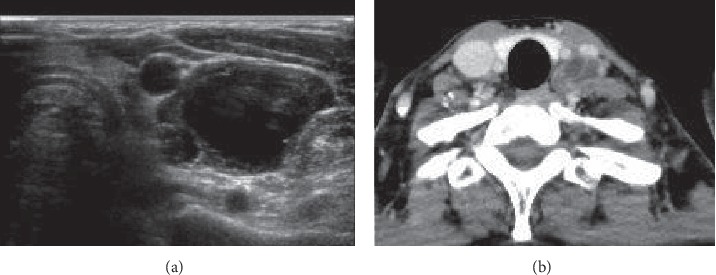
Left lateral cervical lymph node imaging. Neck ultrasonography (a) and CT scan (b) images showing cervical lymph node swelling.
